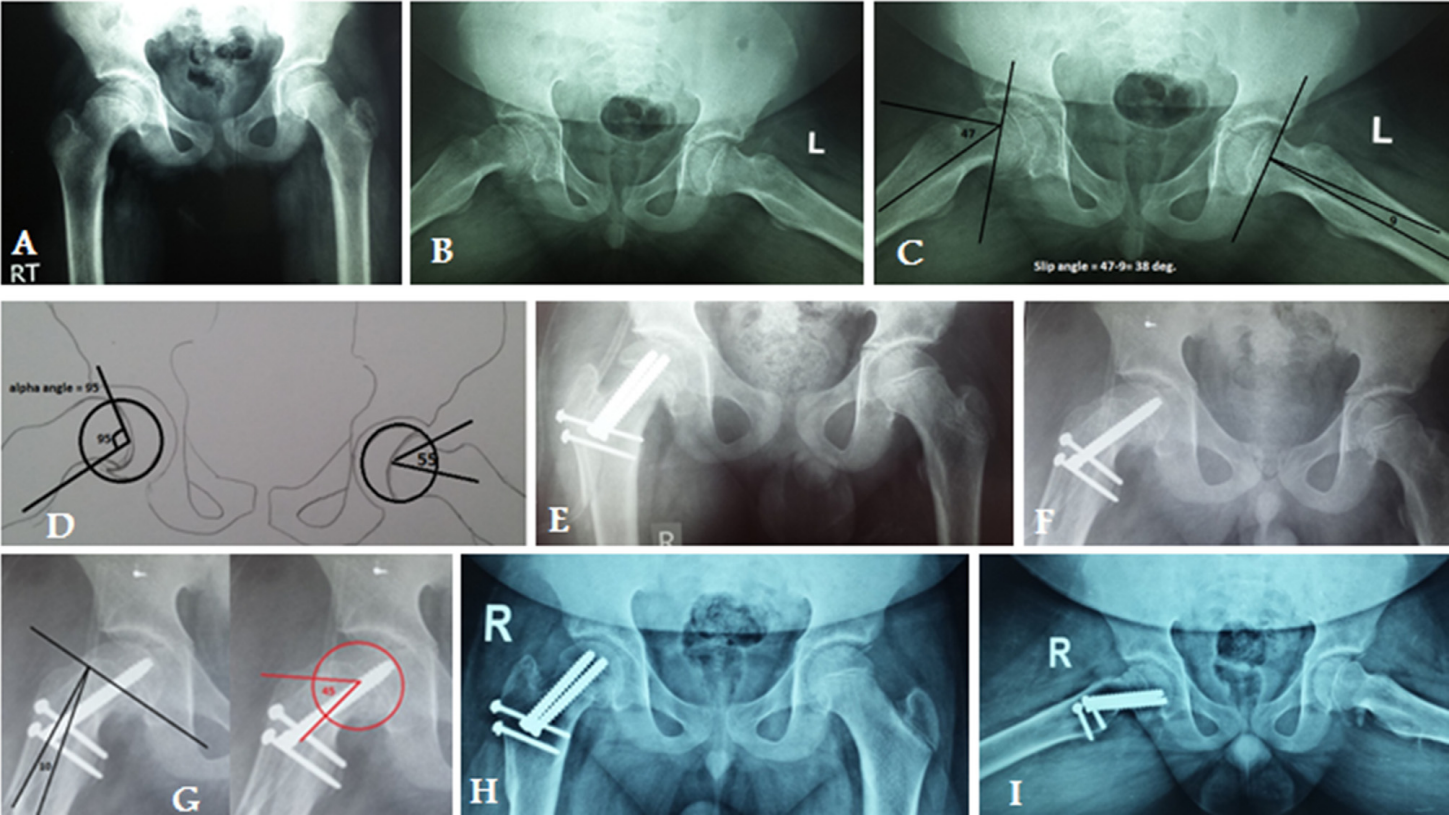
X-ray in 15-year-old male with Rt chronic stable SCFE (case number 4): (A) preoperative AP pelvis, (B) preoperative frog lateral view of both hips, (C) preoperative measurement of Southwick slip angle, (D) preoperative measurement of the alpha angle, (E) and (F) postoperative AP and frog view, (G) postoperative measurement of Southwick slip and alpha angles, (H) 12-month postoperative AP and frog lateral X-ray showing complete union of trochanteric osteotomy and physis with no evidence of AVN.
